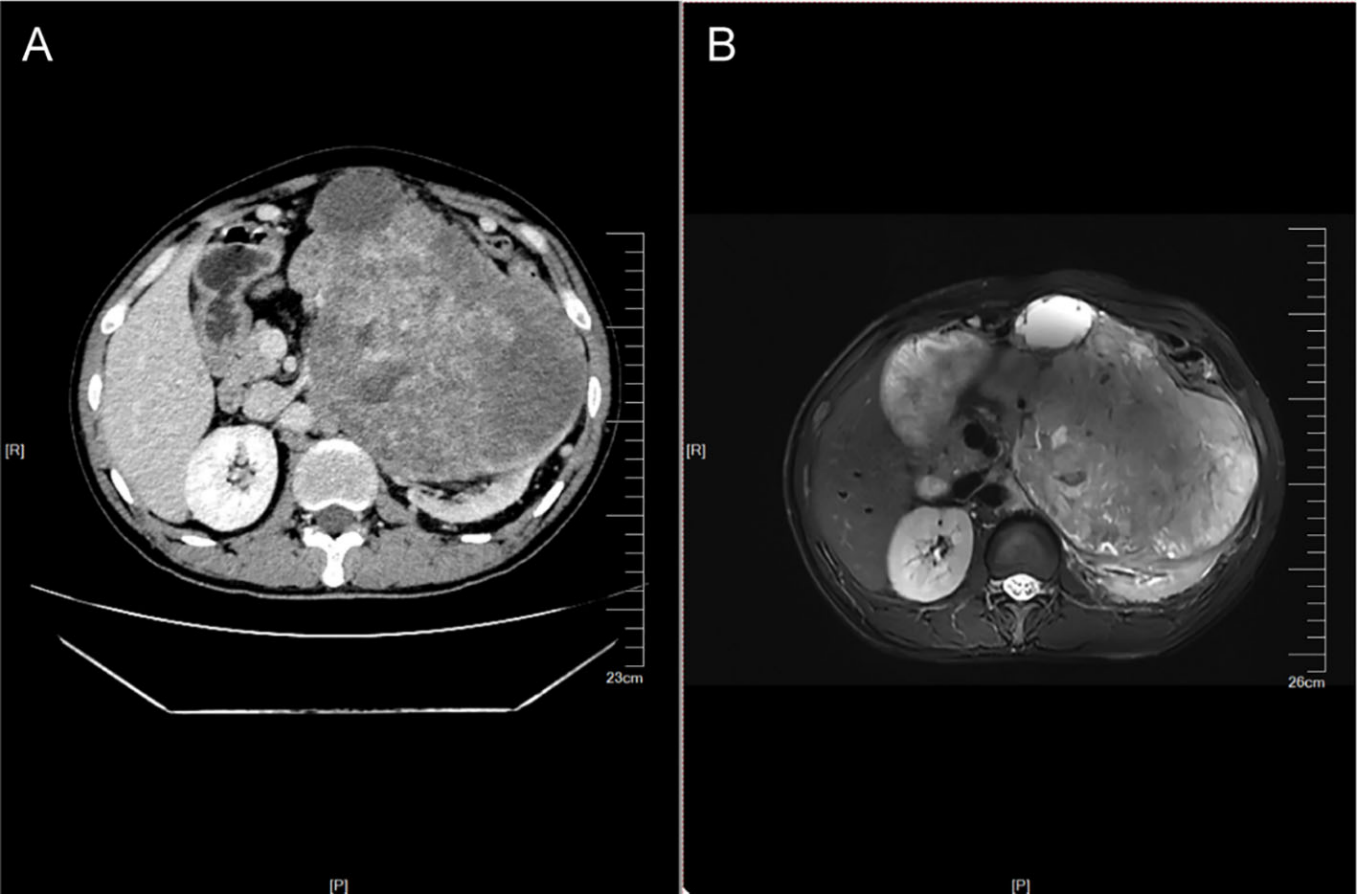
Preoperative imaging examination. (A) Contrast-enhanced CT images show the morphology of the mass. (B) MRI images show the morphology of the mass.
